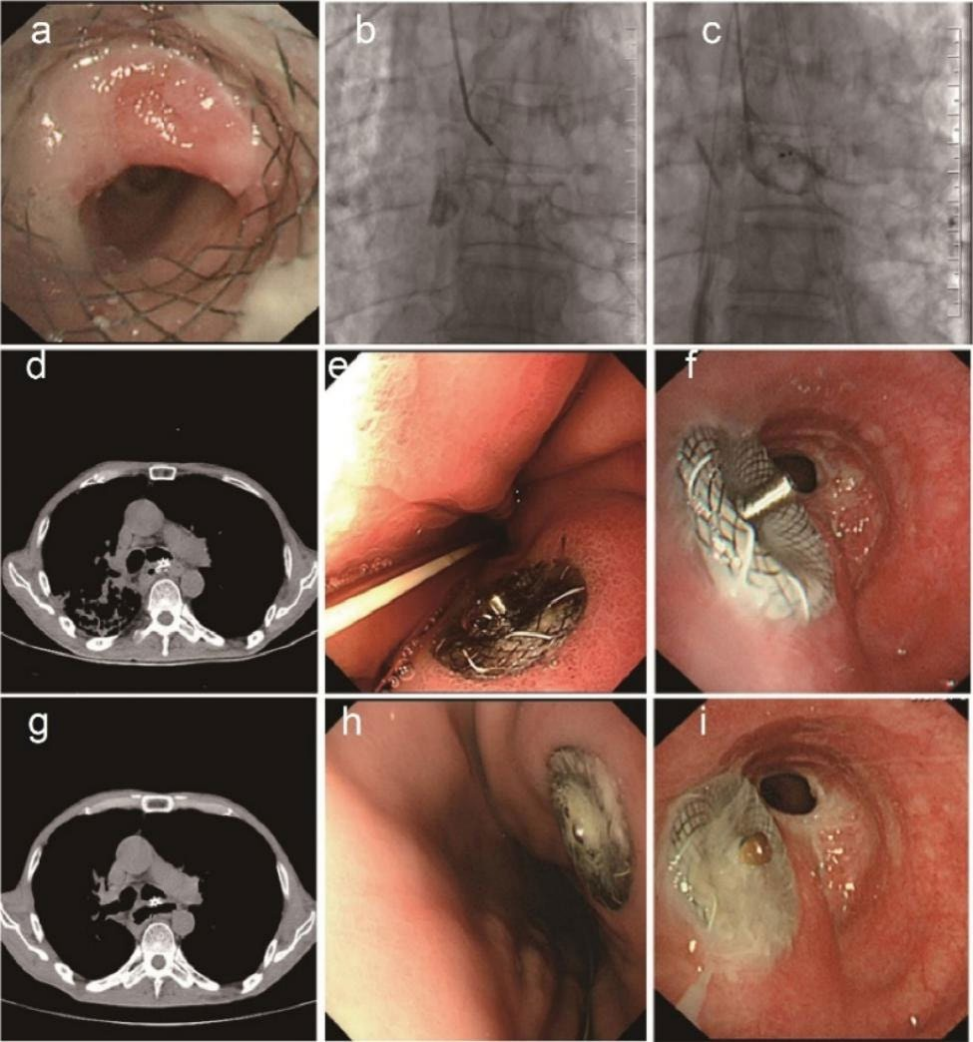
ASD placement to treat a GBF the failed to be cured by a covered Y-shaped self-expandable metallic stent. The covered Y-shaped self-expandable metallic stent was removed due to granulation hyperplasia (a). Bronchogram demonstrated that the GBF was not cured by the stent (b), and an ASD was placed to occlude the GBF (c). Representative images of ASD-occluded GBFs from CT (d), gastroscopy (e), and bronchoscopy (f). Representative images of ASD from CT (g), gastroscopy (h), and bronchoscopy (i) 6 months after placement
